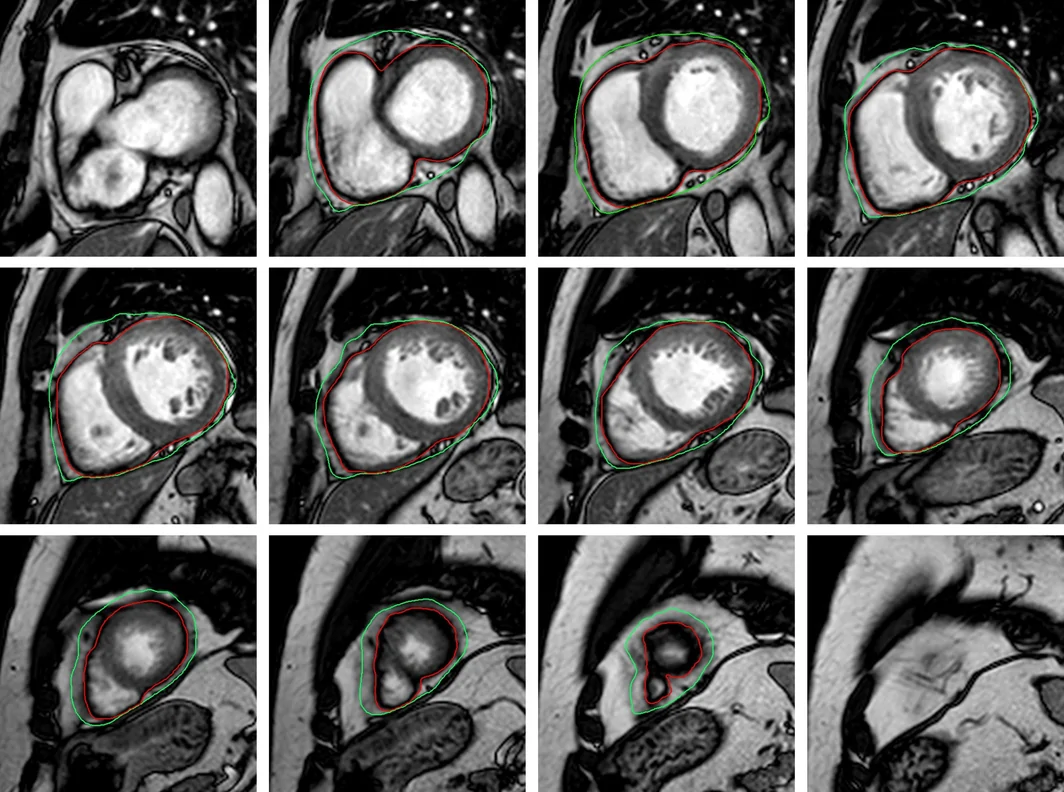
MRI‐derived quantification of epicardial adipose tissue using a short‐axis balanced steady‐state free precession cine sequence. The epicardial adipose tissue is located between the green line following the parietal layer of the serous pericardial border and the red line, which marks the epicardium (the visceral layer of the serous pericardium).
